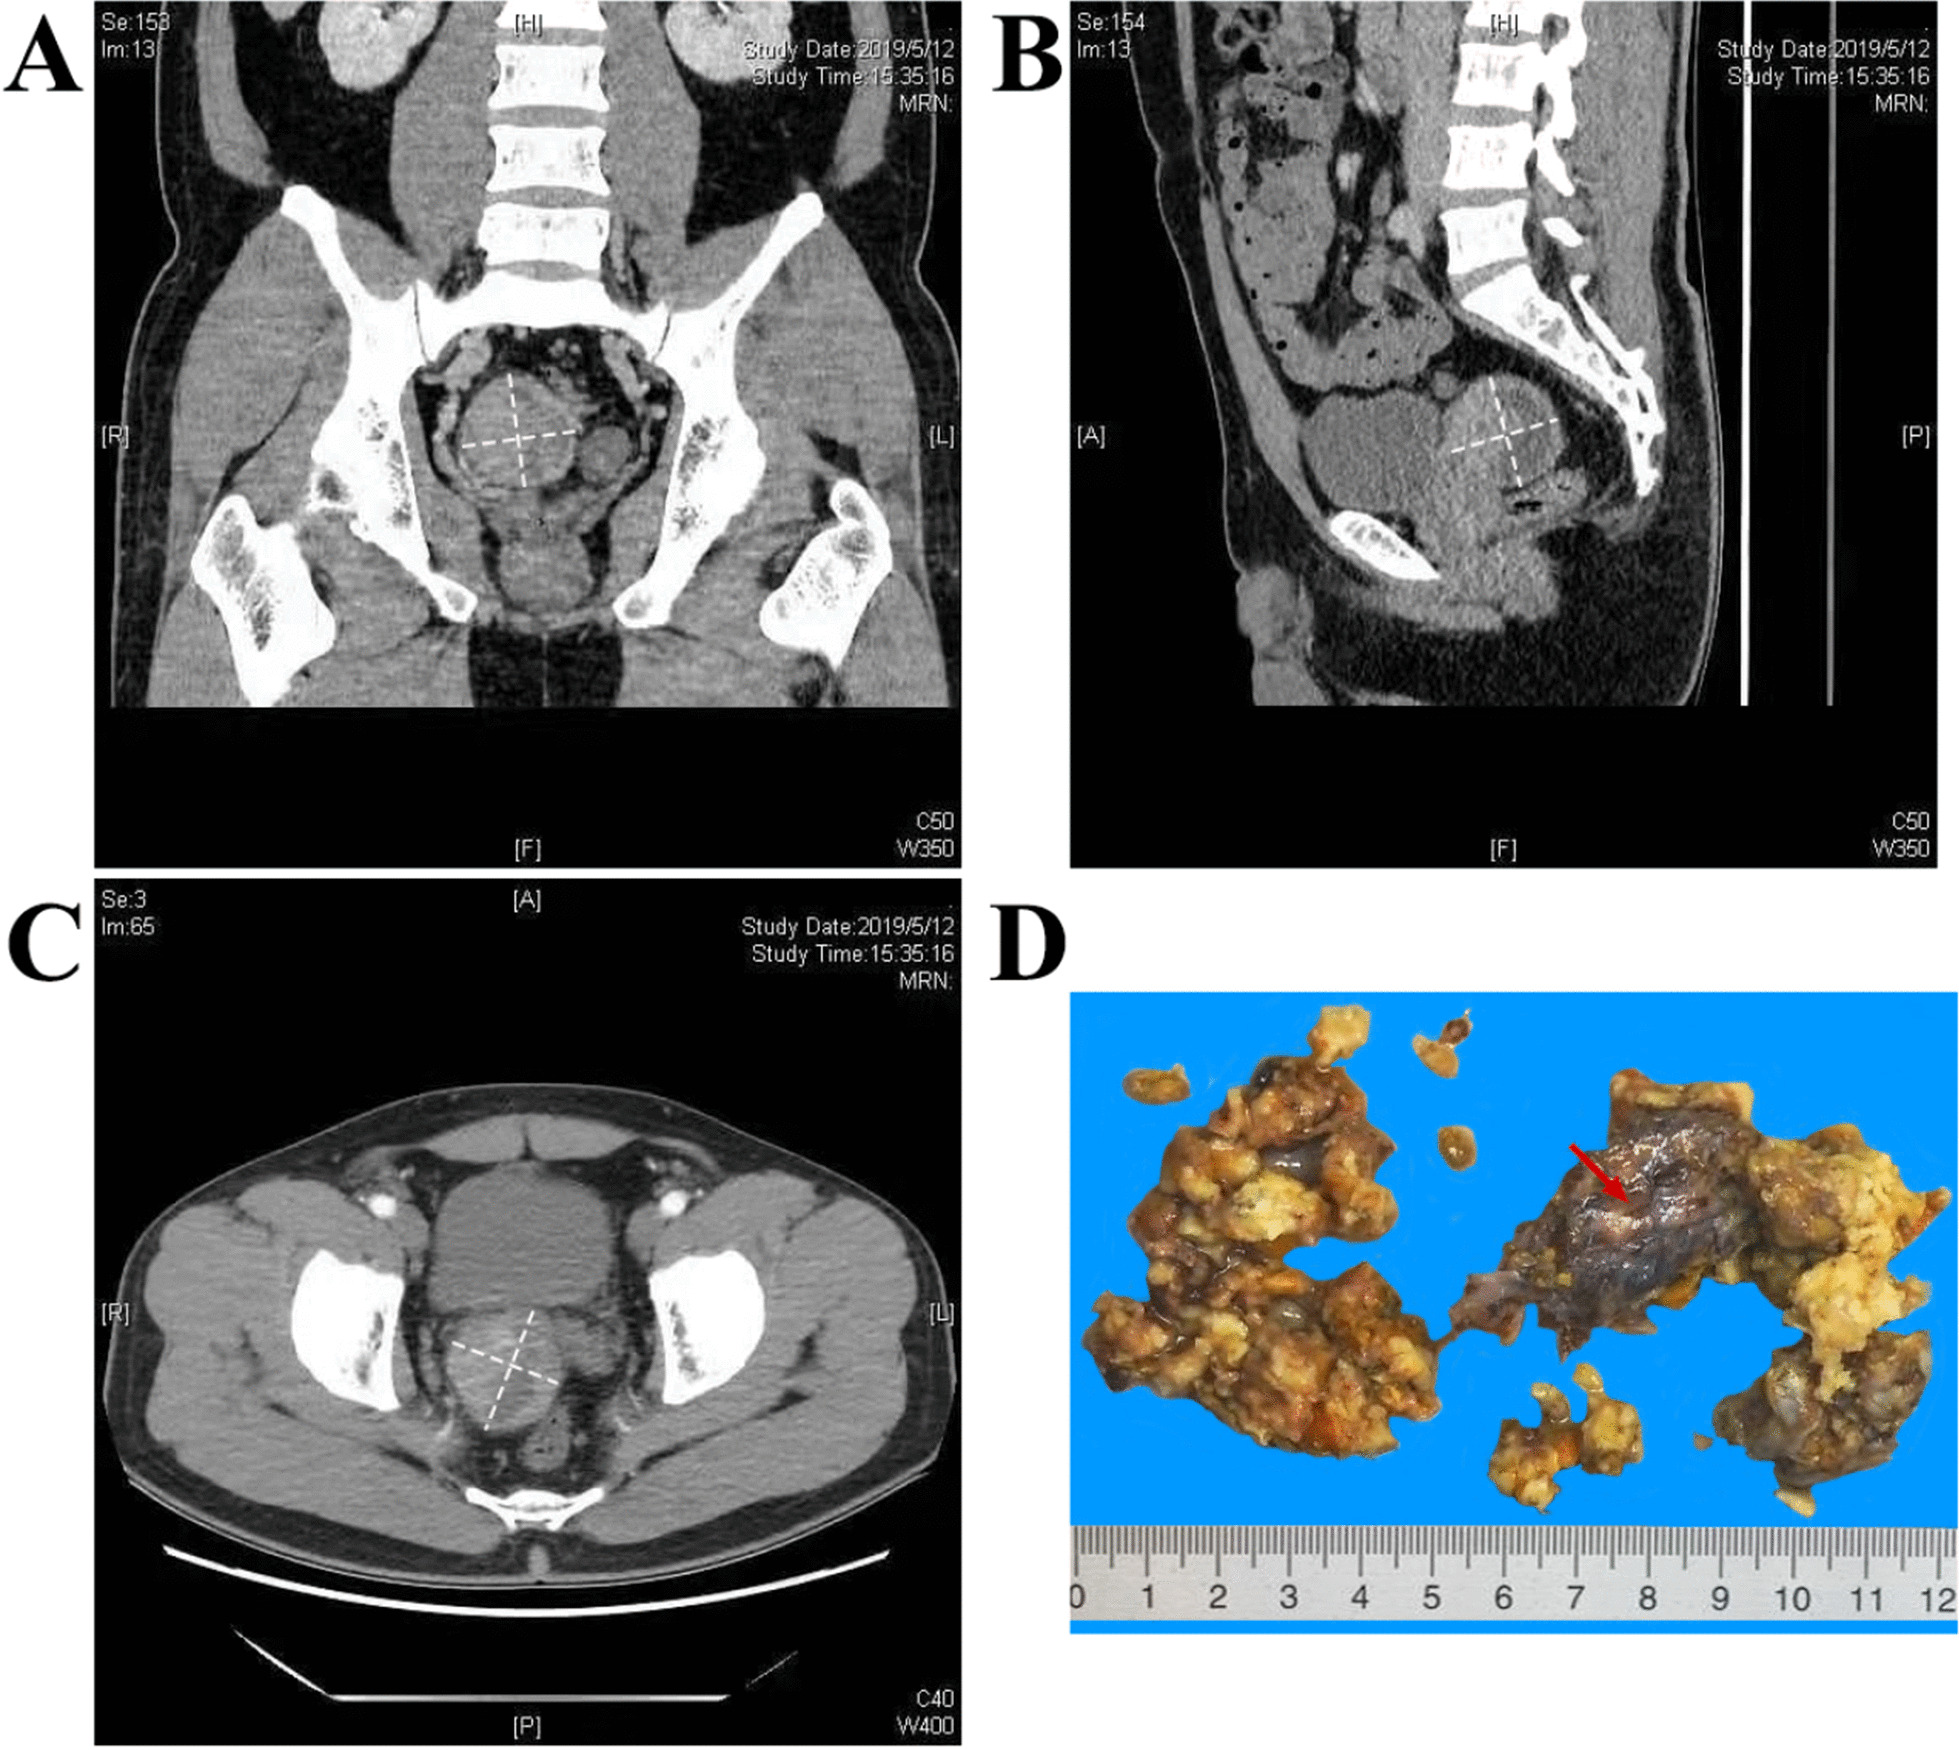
Clinical imaging findings. a–c CT examination revealed a mixed density mass with a clear boundary. d Macroscopically, the tumor was an 80 × 55 × 2-mm mass with a fibrous capsule (red arrows)
